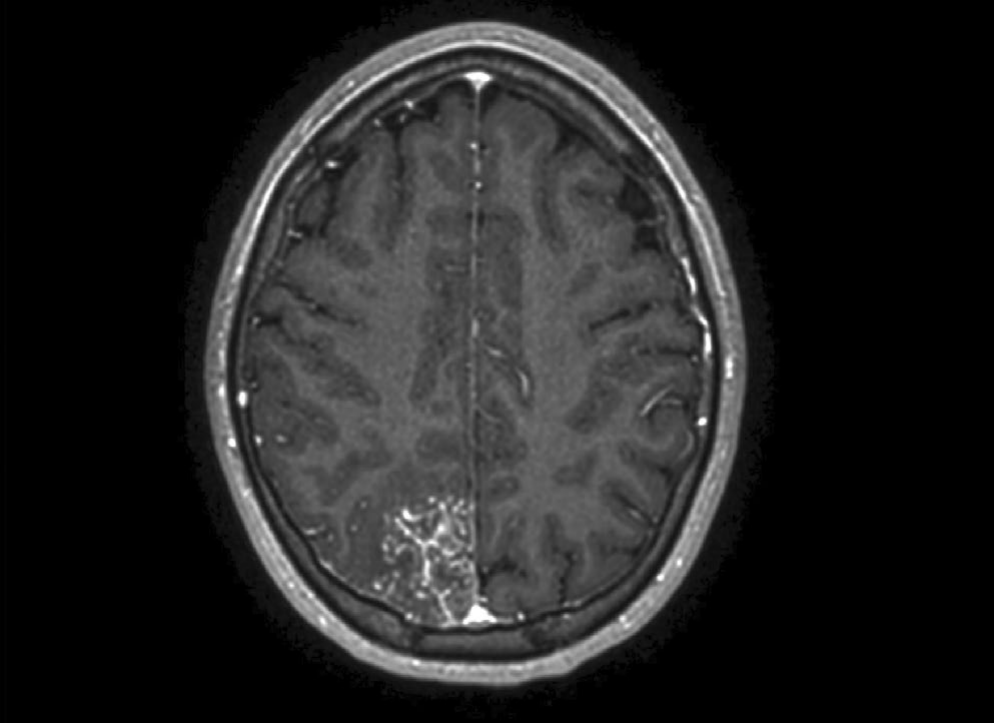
FIGURE 2 Brain MRI in axial T1
